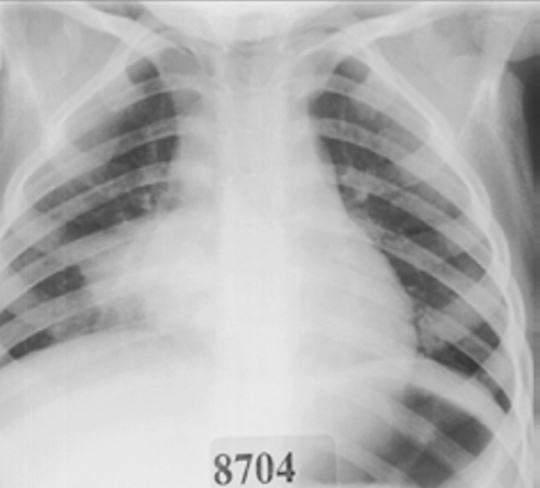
Chest radiograph showing right lower lobe pneumonia
